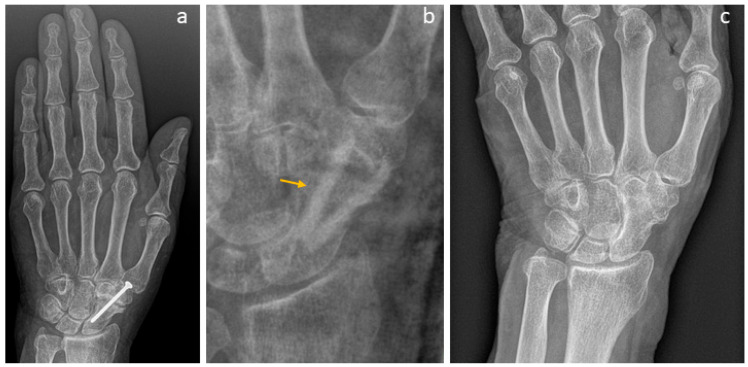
A patient was treated to overcome the non-union of the scaphoid–trapezoid–trapezium arthrodesis. (a): pre-revision X-ray; (b): the metal screw was removed, and the patient was treated with two Shark Screws^®^ (orange arrow)—union was detected eight weeks after revision surgery. (c): 14 months after revision, the Shark Screw^®^ is no longer visible.
